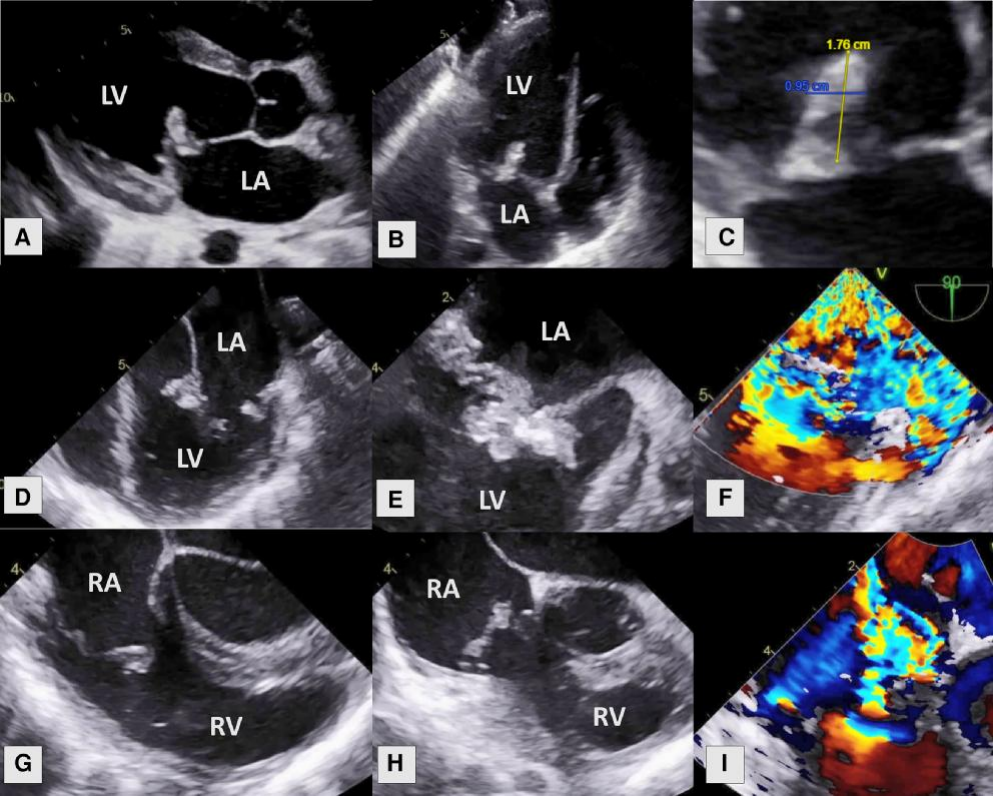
Transthoracic and transoesophageal echocardiogram view of MV and TV vegetations. (*A*) Parasternal long-axis view revealing large MV vegetation; (*B*) apical four-chamber view; (*C*) zoomed in on vegetation measuring 9.5 × 17.6 mm; (*D*) mid-oesophageal four-chamber view of large MV vegetation; (*E*) zoomed in on MV in end-diastole; (*F*) severe mitral regurgitation on colour Doppler; (*G*) mid-oesophageal right ventricle inflow–outflow view of TV vegetation in mid-diastole; (*H*) TV vegetation in mid-systole; (*I*) severe tricuspid regurgitation on colour Doppler. MV, mitral valve; TV, tricuspid valve; LA, left atrium; LV, left ventricle; RA, right atrium; RV, right ventricle.
